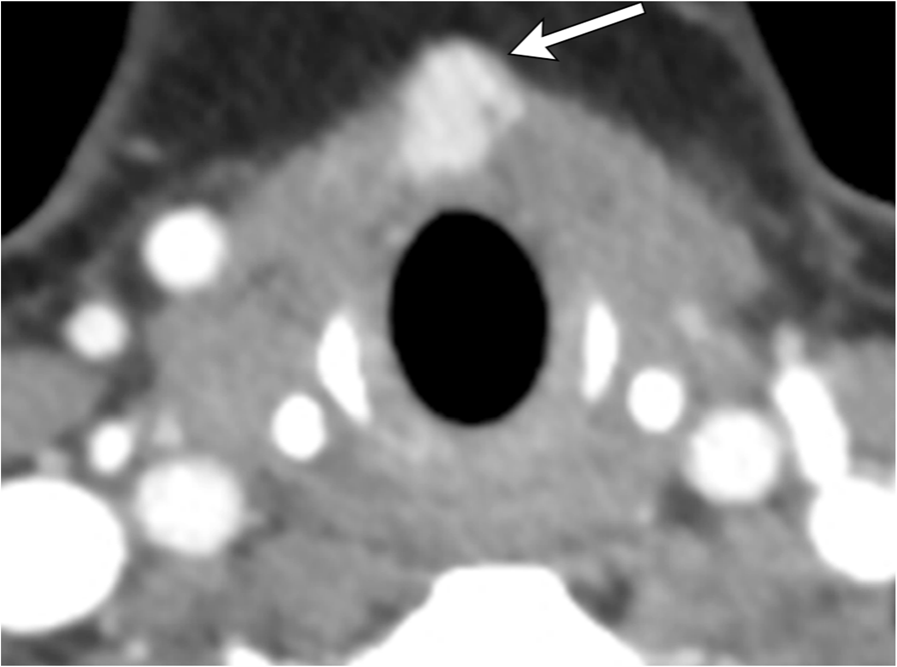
Ectopic thyroid tissue. Axial contrast-enhanced CT image demonstrates a focal high attenuating soft tissue mass in the midline anterior neck along the anterior margin of the thyroid cartilage (arrow). Invaginated by strap musculature, this mass resembles the thyroid gland in attenuation and occurs in expected course of the thyroglossal duct tract
